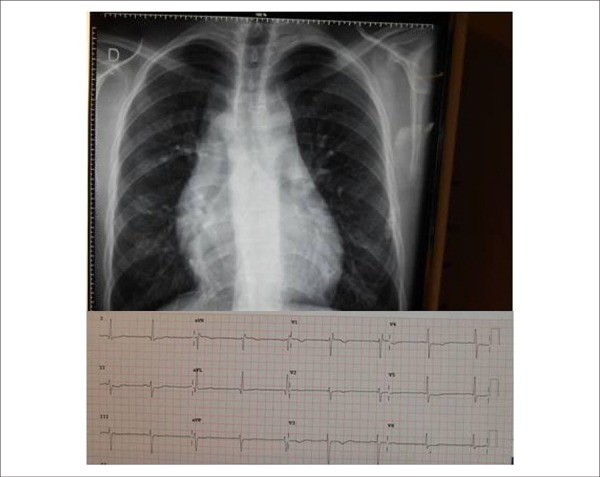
Chest radiograph shows moderate enlargement of the cardiac silhouette.
